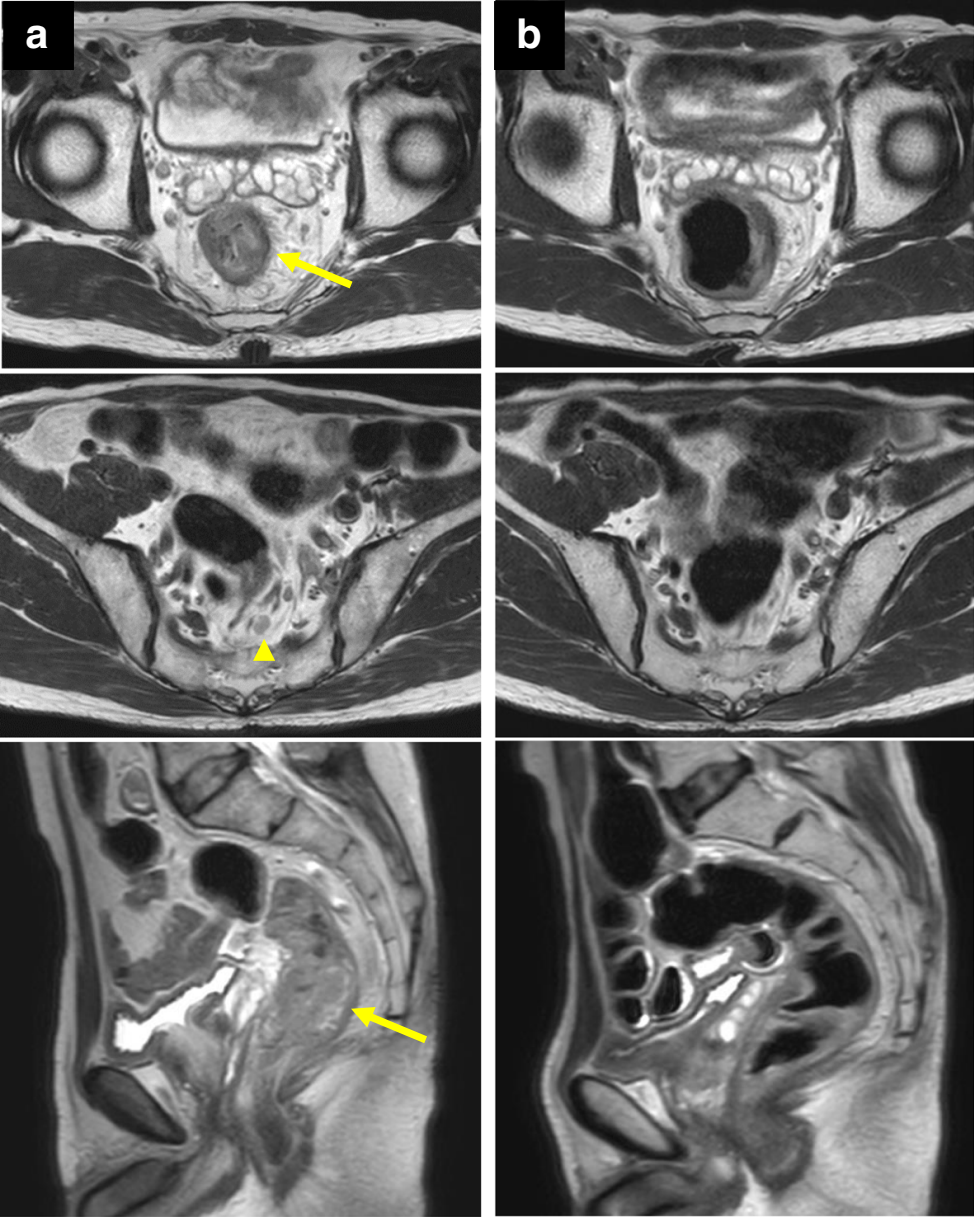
MRI findings. Pelvic MRI showed a large tumor in the rectum (arrow) and a pararectal lymph node swelling (arrow head) (a). Five weeks after chemoradiotherapy, they were remarkably shrunk (b)
